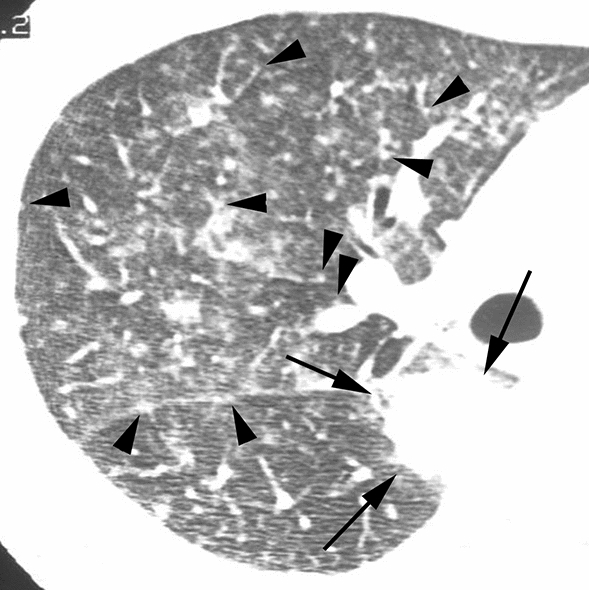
Pulmonary infiltration due to underlying malignancy (leukemic infiltration) in a 44-year-old male patient with acute myelogenous leukemia.HRCT image of the right middle lung showing multiple nodules varying in size. Note that small nodules are attached to the pleura or thickened BVB (arrowheads), with differences in the number of nodules in the segments, reflecting a perilymphatic distribution rather than a random distribution. Note also that a large nodule is observed in the right lower lobe (arrows)
